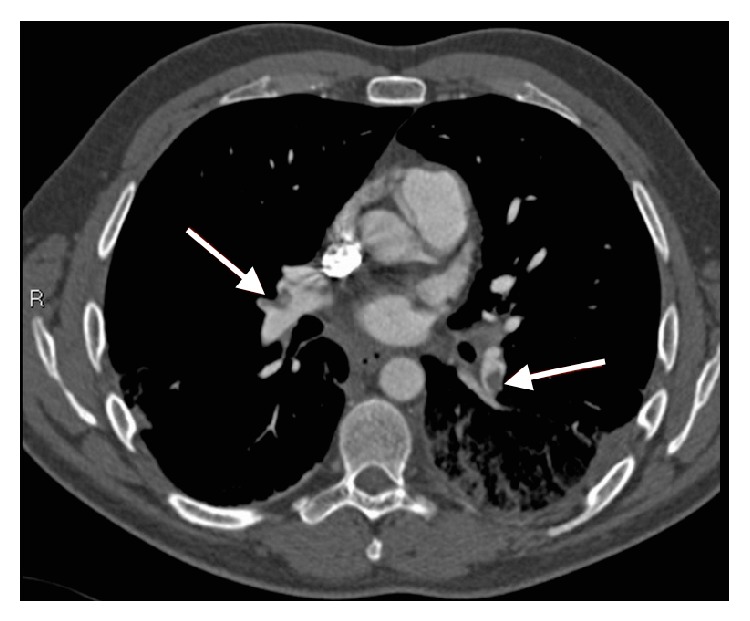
CT pulmonary angiography (CTPA) showinga bilateral pulmonary embolisms with clots in branch arteries.
